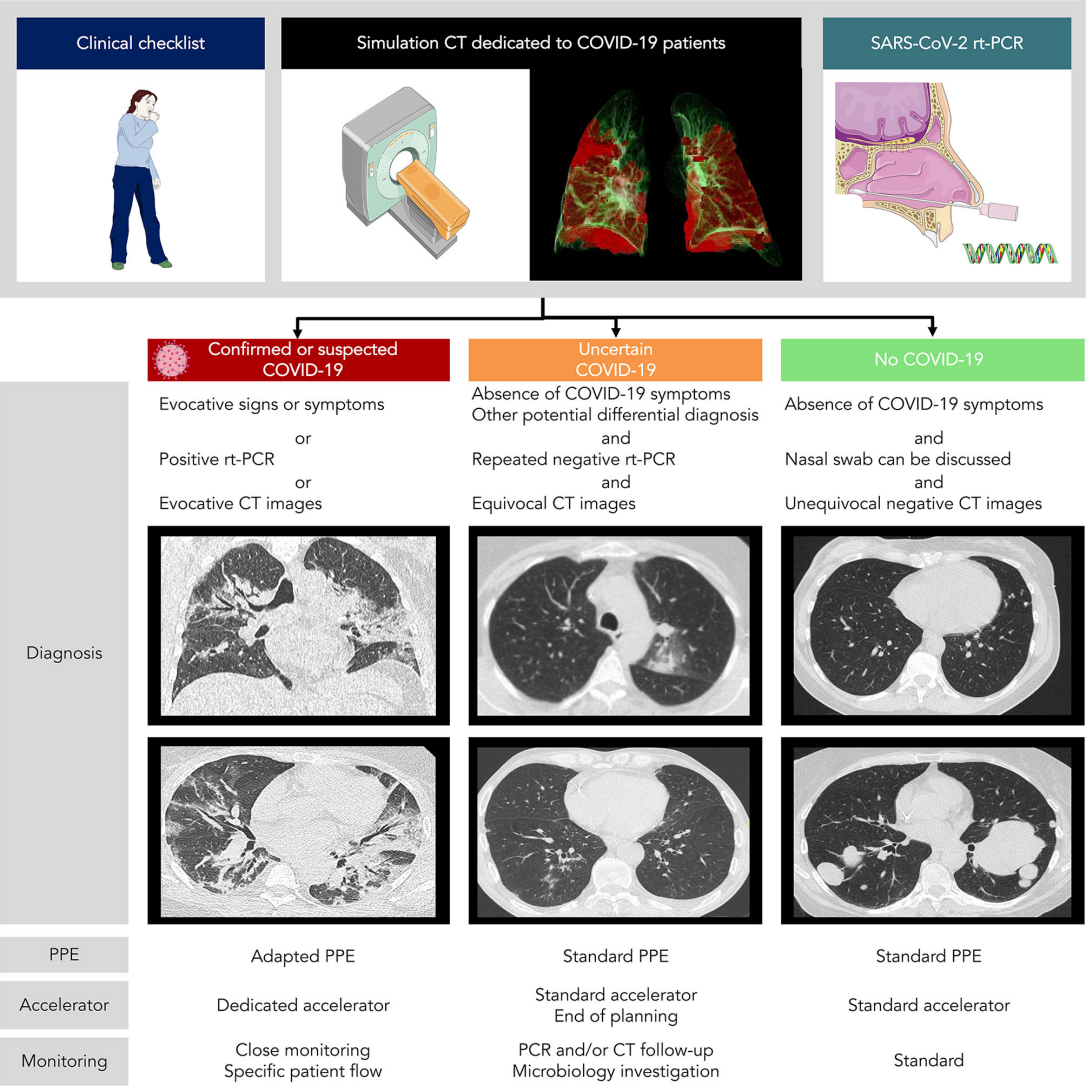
COVID-19 patients management in radiotherapy according to chest CT + rt-PCR screening. PPE, personal protective equipment.
